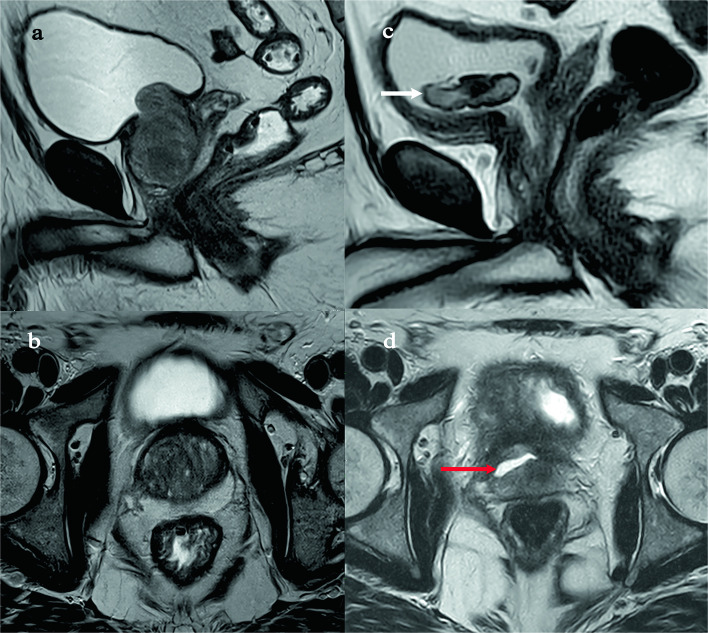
Prostate MRI before and after prostatic artery embolization. Preprocedural sagittal (a) and axial T2-weighted images (b) showing benign prostatic enlargement with nodules in the transition zone and intravesical protrusion of the median lobe. c, d Three-month follow-up MRI. Prostate volume had decreased to 15 cm^3^, corresponding to a 73% reduction. c A large intravesical mass is visible (white arrow), initially interpreted as clot retention. d Central cavitation (red arrow) with regression of the median lobe, resembling a transurethral resection cavity
